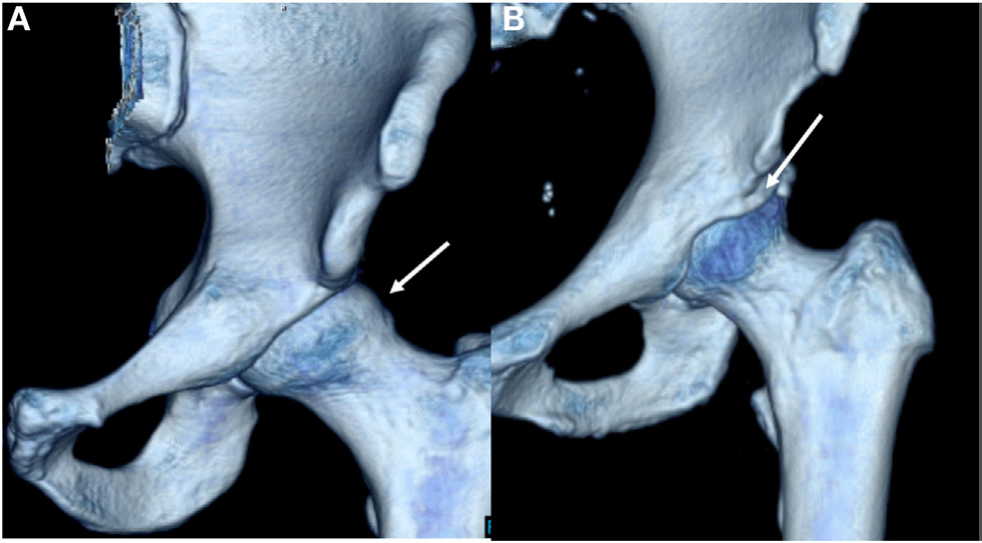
Three-dimensional CT reconstructions demonstrating cam (A) and pincer (B) deformities.
